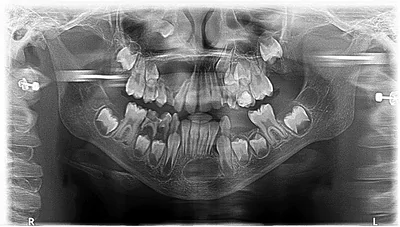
Panoramic radiograph showing the presence of two cysts: one in the mandible and one in the maxilla.
